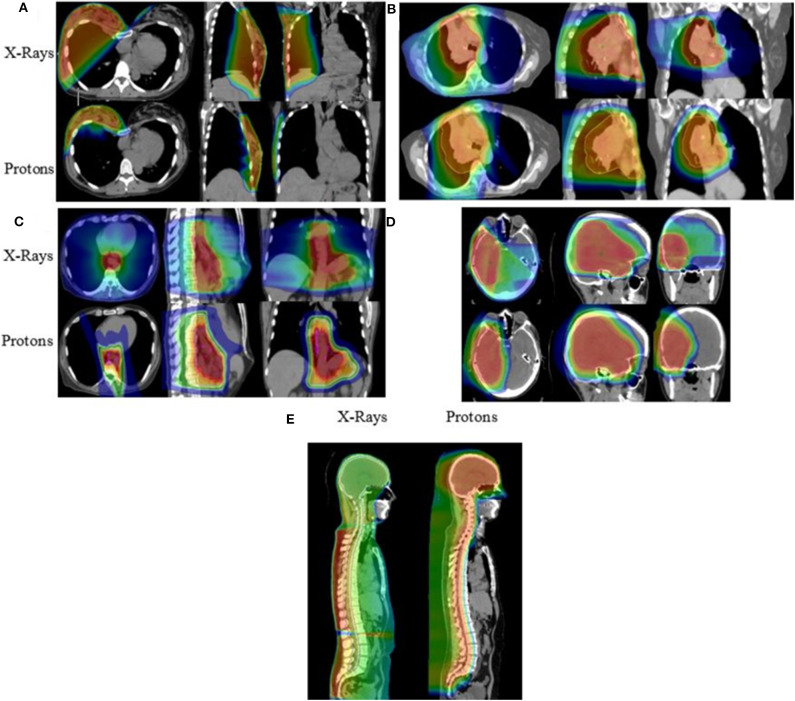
Isodose distributions of the proton radiotherapy plans with comparison x-ray plans generated for patients treated with PBT including patients with (A) breast cancer, (B) locally advanced NSCLC, (C) esophageal cancer, (D) primary low-grade glioma, and (E) ependymoma receiving craniospinal irradiation.
